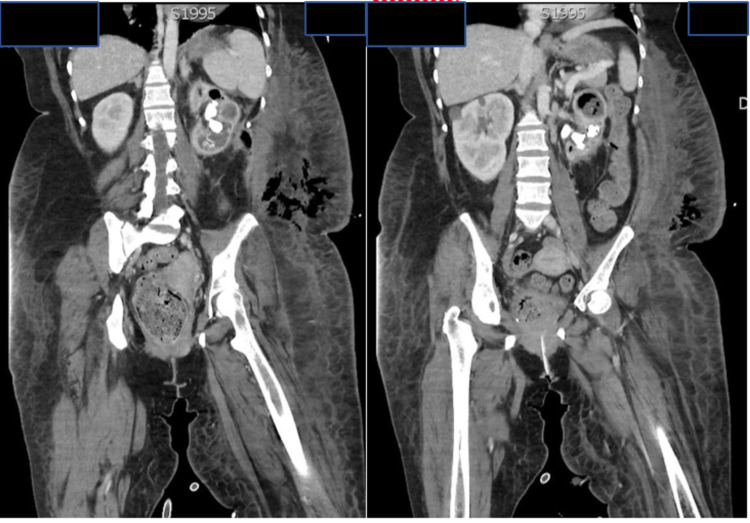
CT abdomen pelvis: large left staghorn calculus with obstructed infected left kidney with emphysematous left pyelonephritis with soft tissue gas in the left retroperitoneum and left flank/posterolateral soft tissues in keeping with necrotising fasciitis.
